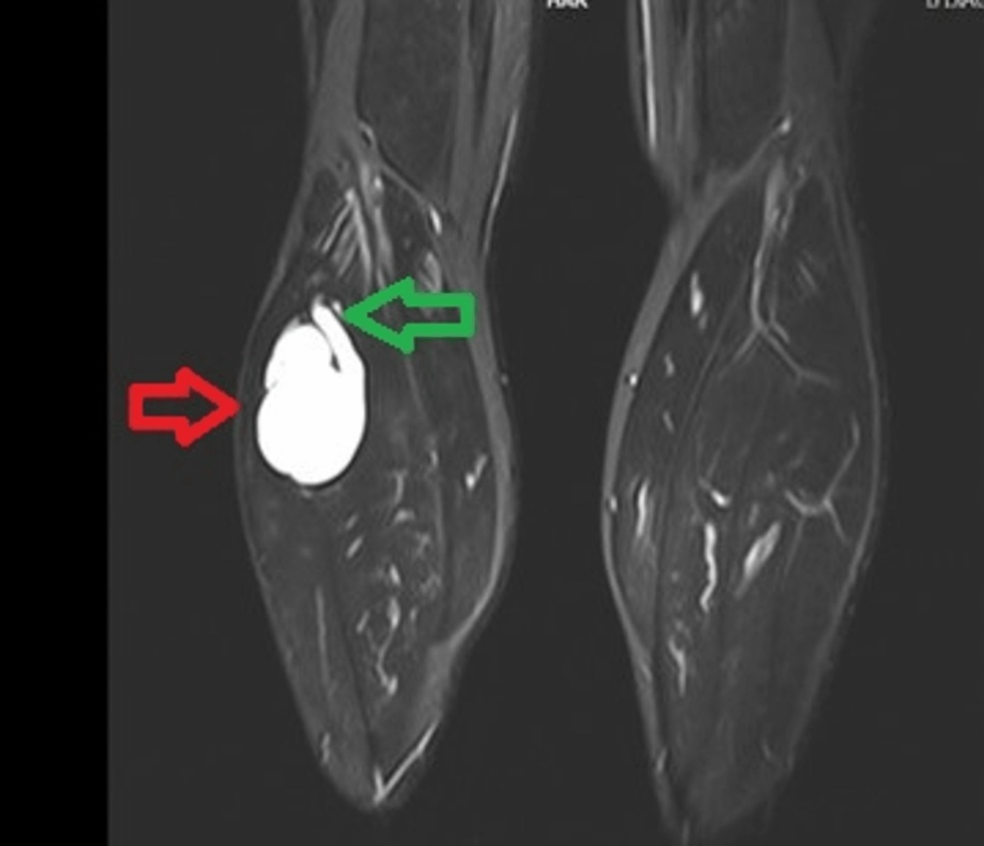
Coronal T2-weighted MRI indicates the lesion (red arrow) and the pedicle (green arrow) arising from the proximal tibiofibular joint. MRI: magnetic resonance imaging
